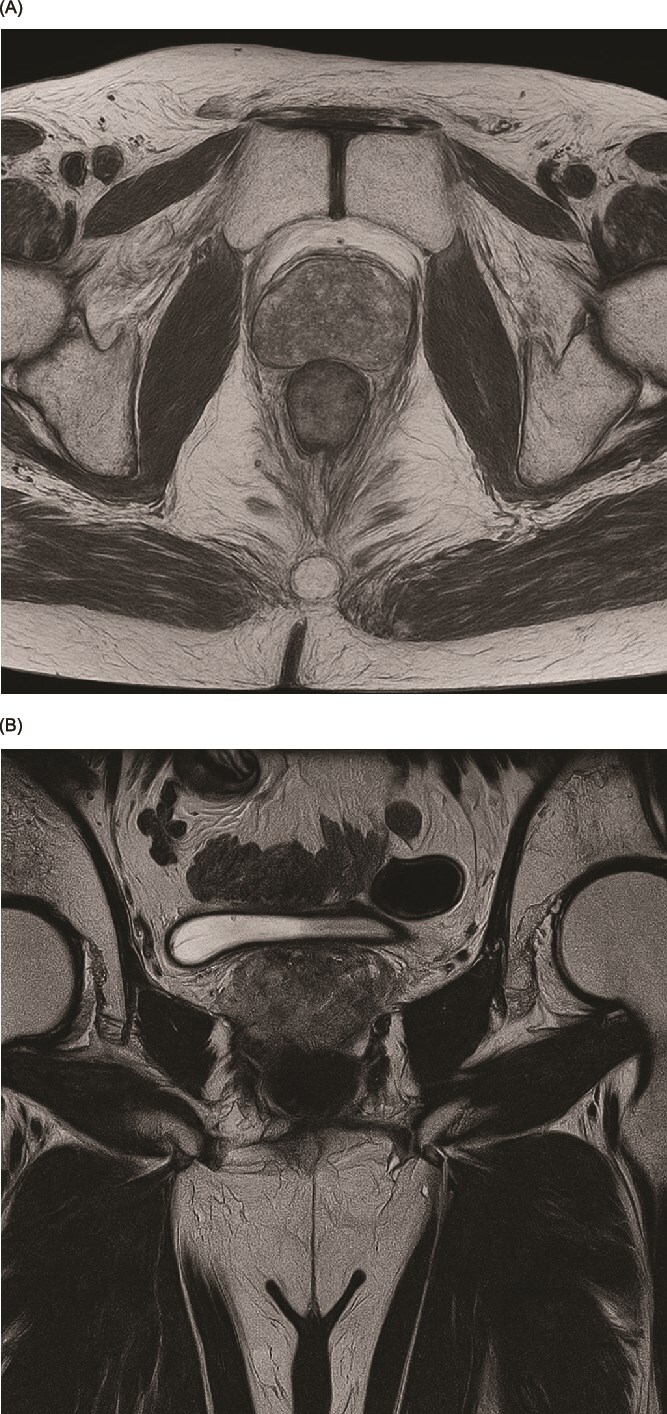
(A, B) Preoperative magnetic resonance imaging (MRI) of the prostate in T2-weighted sequence demonstrating the lesion. (A) Axial view. (B) Coronal view.
